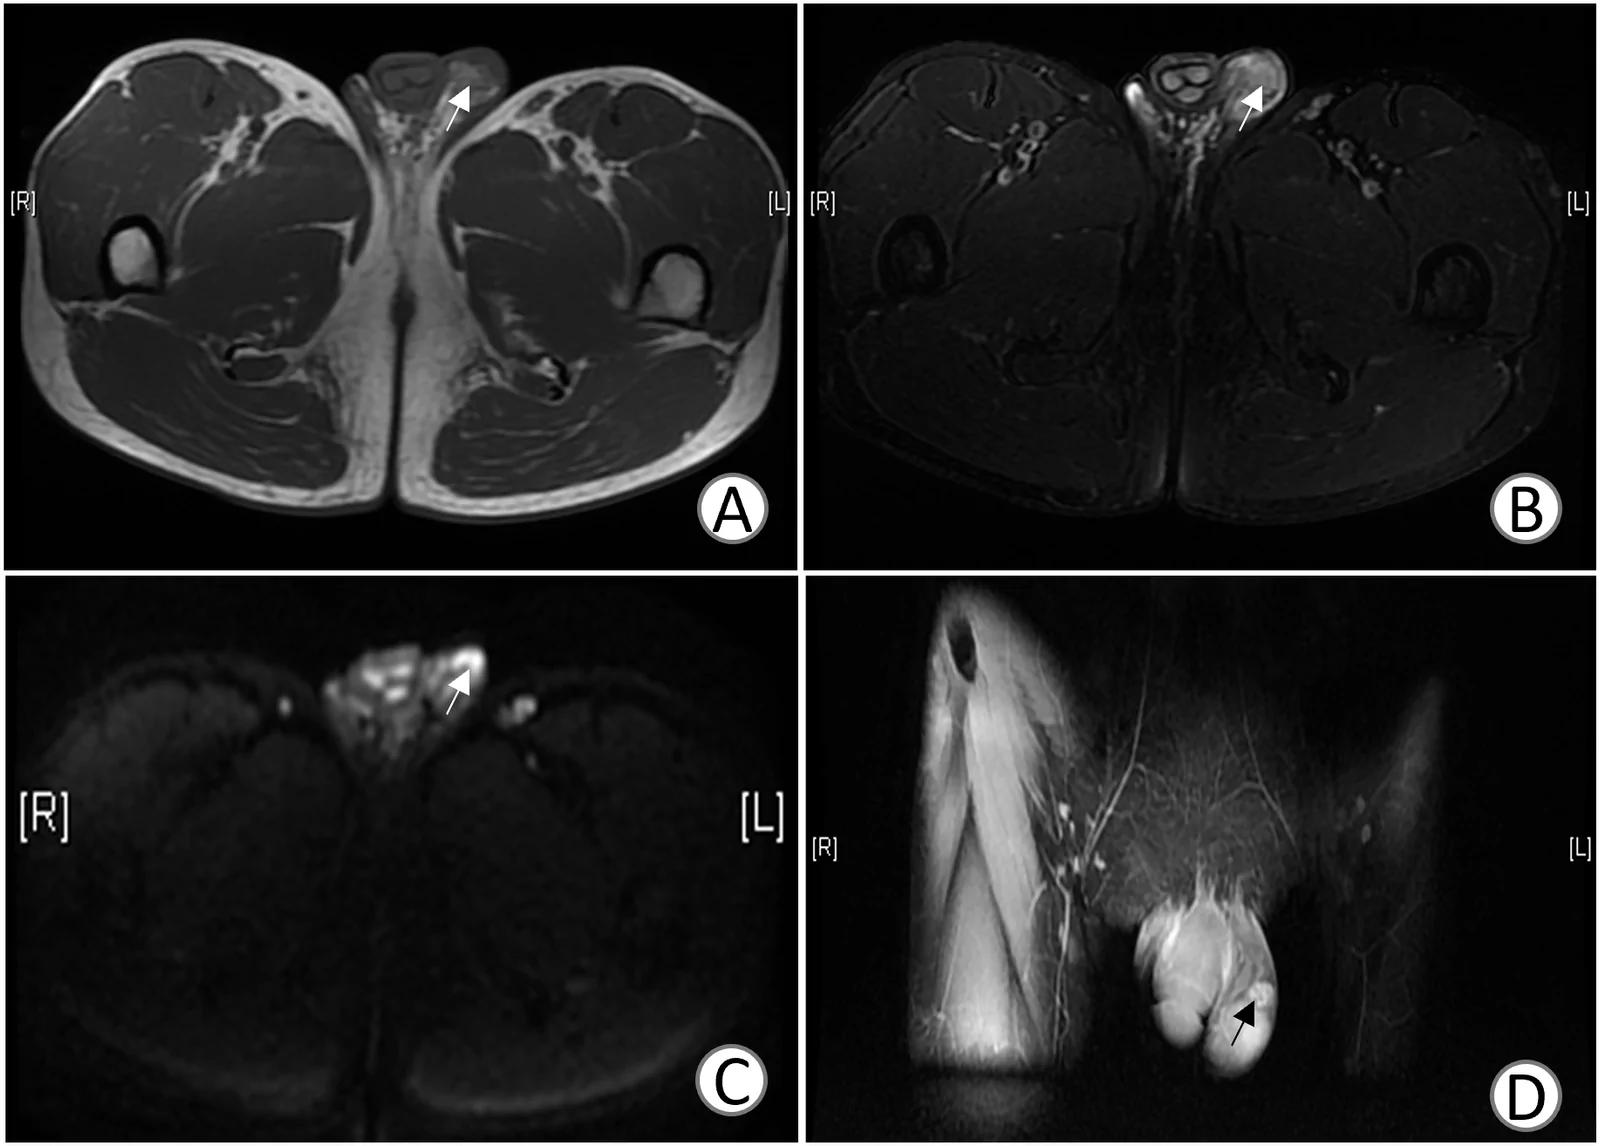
MRI features of primary testicular intestinal-type adenocarcinoma. (A) Axial T1-weighted image shows a hypointense nodule in the upper portion of the left testis. (B) Axial T2-weighted image shows corresponding hypointensity. (C) Axial diffusion-weighted image shows high signal intensity, consistent with restricted diffusion (D) Coronal fat-suppressed contrast-enhanced T1-weighted image shows marked peripheral-dominant enhancement.
